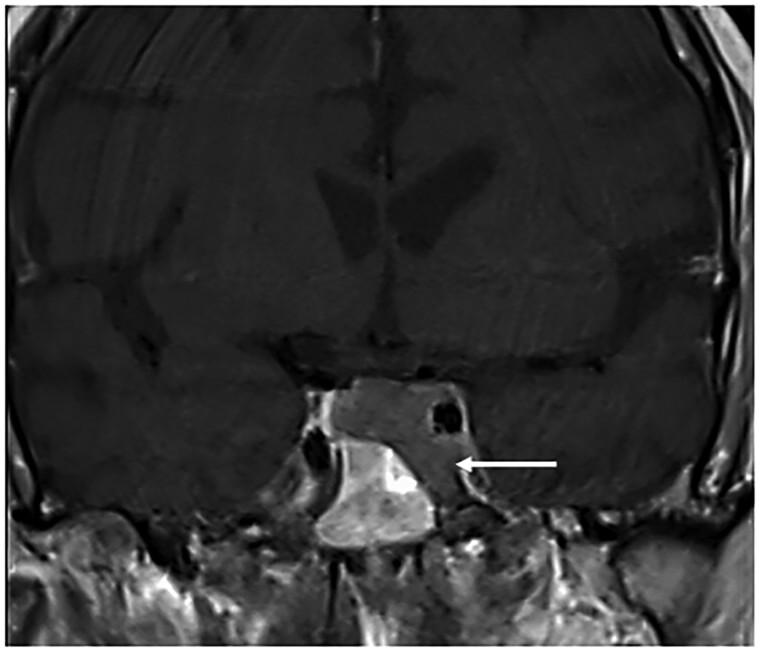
Pituitary magnetic resonance imaging (MRI) with gadolinium, using T1-weighted, turbo spin-echo revealed sequence revealed a 16 × 20 × 16 mm macroadenoma invading the left cavernous sinus (white arrow).
